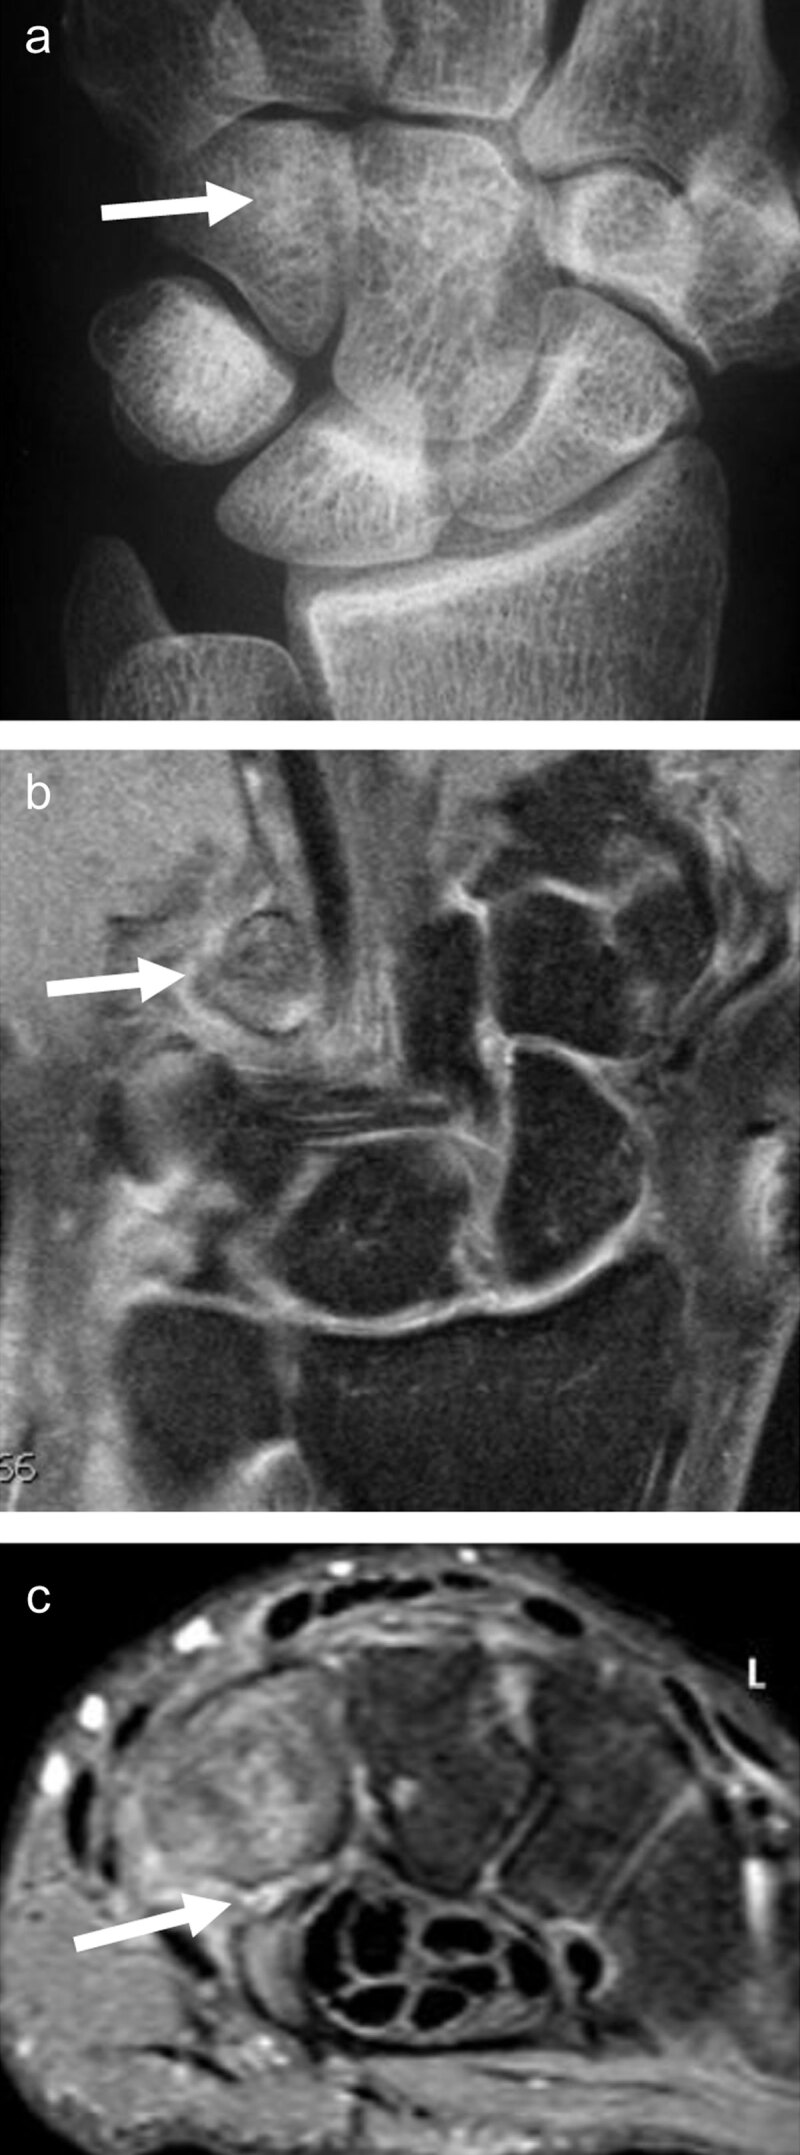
Fracture of the hook of the hamatum, easily missed on conventional radiographs and on fat-saturated MR images. The AP X-ray (a, arrow) demonstrates the absence of the ring sign (normally present at the level of the hamulus) on the hamate bone. On palmar proton-density fat-saturated coronal image (b, arrow), the presence of BME within the hamulus may be obscured because of the close signal intensity of the surrounding muscles. The fracture line at the base of the hamulus could also be missed on the axial proton-density fat-saturated sequence (c, arrow).
